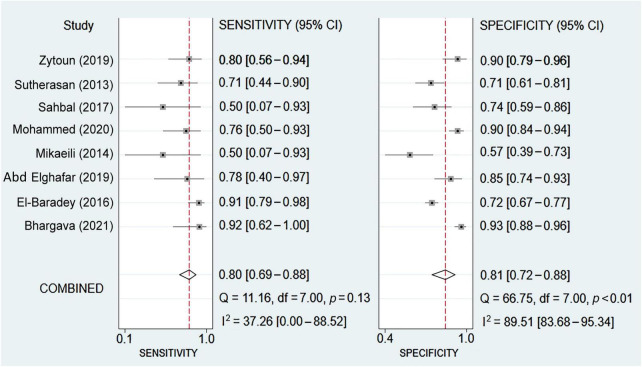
Forest plot showing the pooled sensitivity and specificity of sonography-measured air column width difference (ACWD) in predicting post-extubation stridor (PES).
